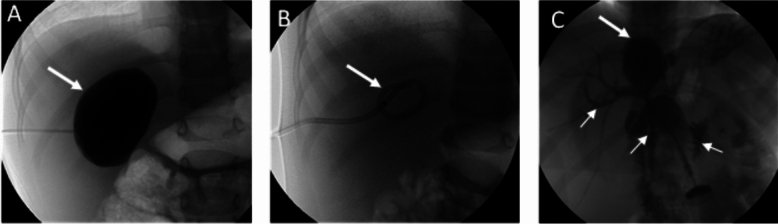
Examples of percutaneous interventions performed on Gharbi Type 1 cysts, categorized ultrasonographically. Images obtained under fluoroscopy during the percutaneous radiologic intervention: A. Cystogram (no cystobiliary fistula—the contrast material administered into the cyst marked with an arrow only filled the cyst without passing into the biliary system); B. Cystogram after the catheter was inserted into the cyst; C. Cystogram after the catheter was inserted into the cyst; C. Cystogram (the contrast medium introduced into the cyst marked with a thick arrow is seen to pass through the intrahepatic biliary ducts and common bile duct marked with thin arrows to the intestinal system (cystobiliary fistula present)
